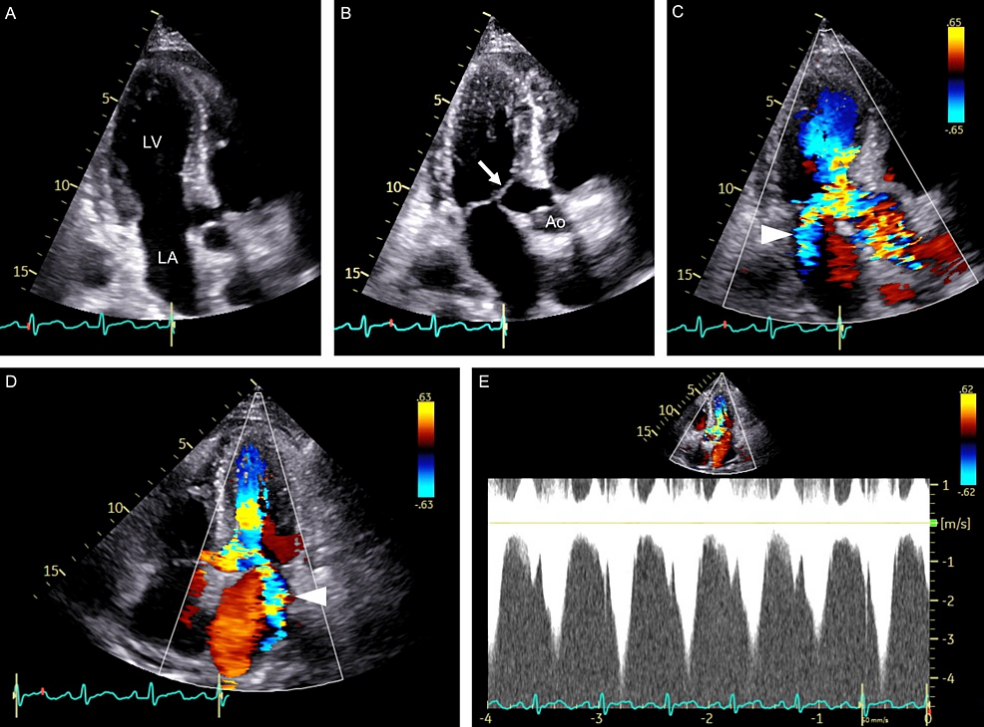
Echocardiography Apical three-chamber views at end-diastole (A) and end-systole (B) show SAM of the mitral valve (arrow). Color Doppler images of an apical three-chamber view (C) and four-chamber view (D) show an LVOT obstruction, along with mitral regurgitation (arrowheads), with a peak flow velocity of 4.0 m/s (E) Ao: aorta, LA: left atrium, LV: left ventricle
